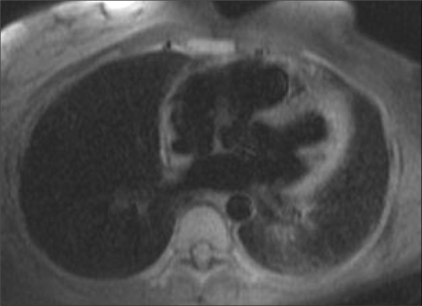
Posttreatment T1W axial MRI image reveals normal appearance of the myopericardium and regression of the mediastinal lymphadenopathy
